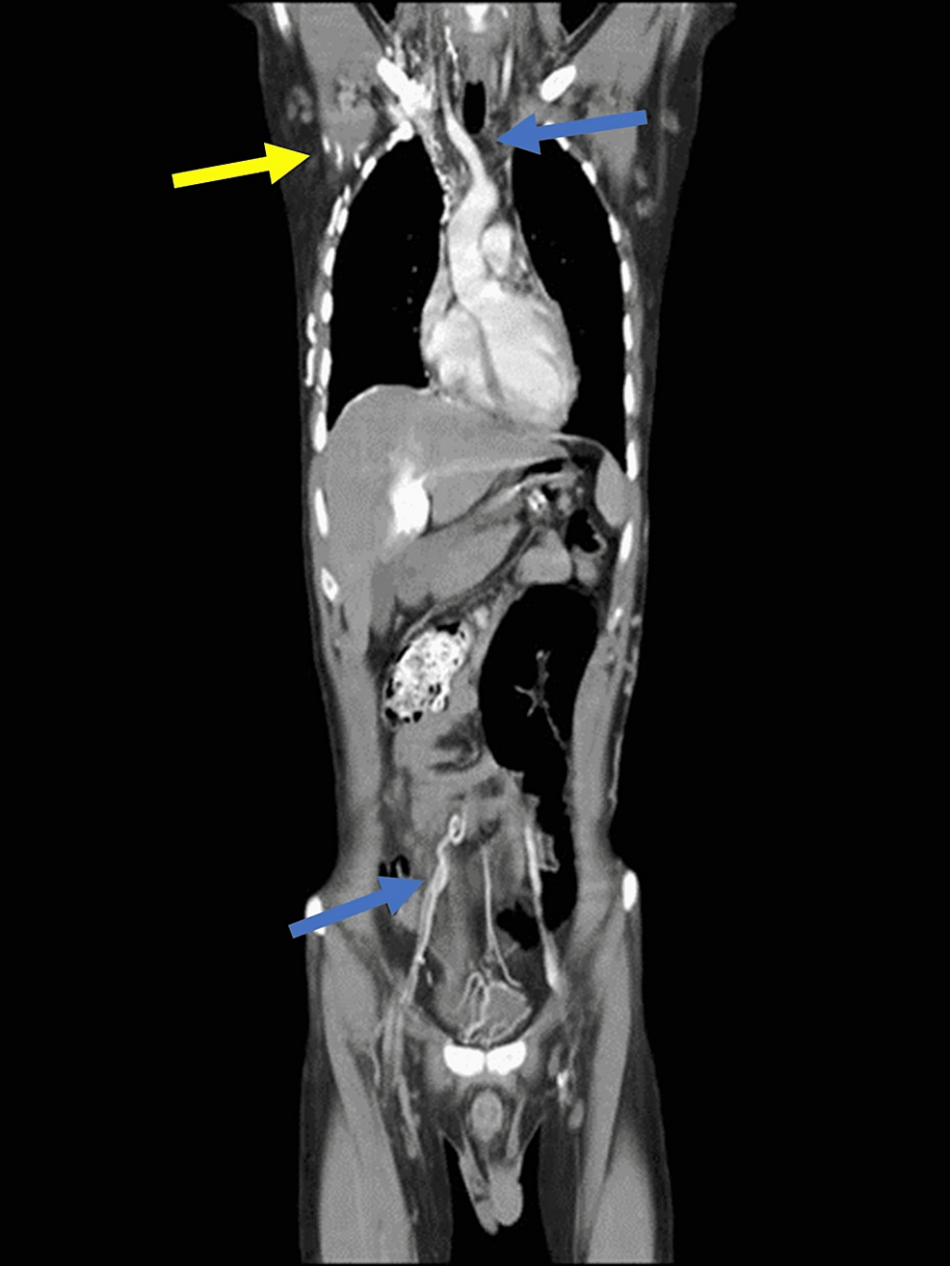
CT CAP showing SVC and right external iliac vein thromboses (blue arrows) and collaterals in axilla (yellow arrow) CT CAP: computed tomography chest abdomen pelvis, SVC: superior vena cava
